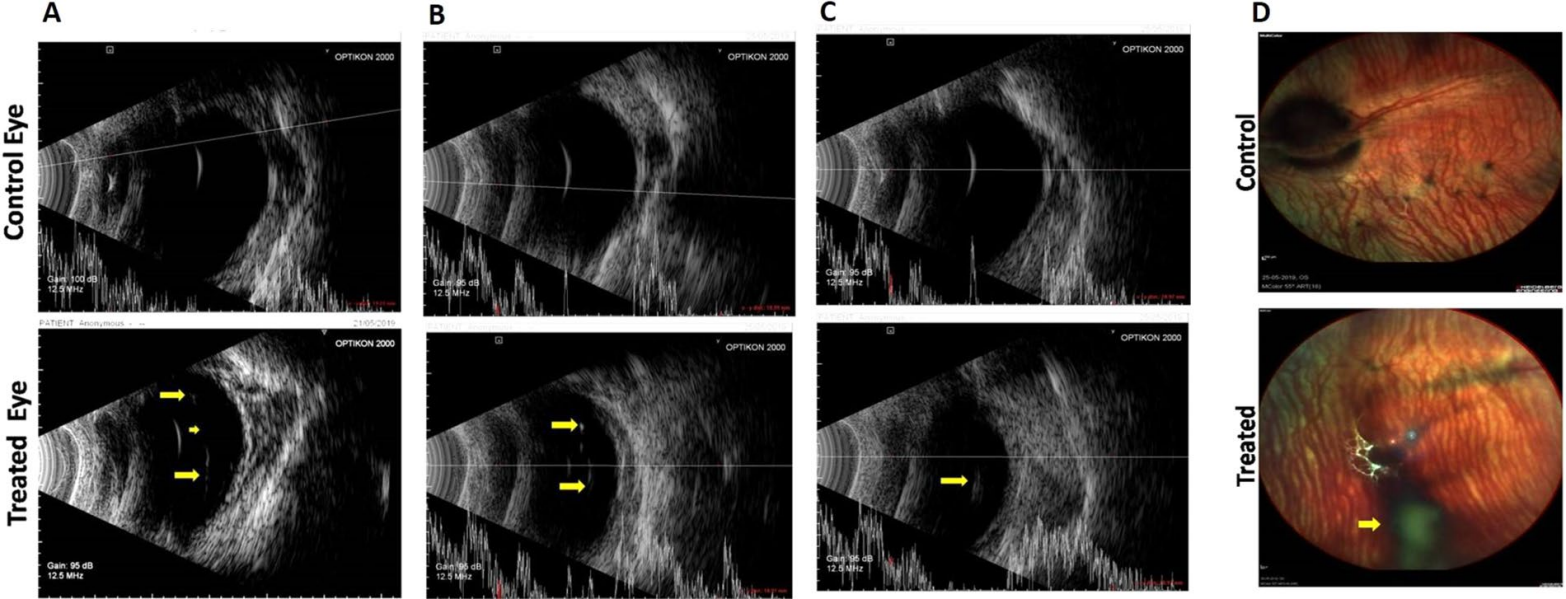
B-scan ultrasonography in three representative eyes following treatment with RCBD + CBD: Representative images of eyes treated with CBD and RCBD showing complete PVD (Panels A and B, marked by arrows), and partial PVD (Panel C), along with the corresponding contralateral eyes (controls). (Panel D) Fundus of representative rabbit eye with complete PVD, following the intravitreal injection of combination of CBD and RCBD; the posterior hyaloid membrane appears as a shadow in the fundus image which reflects PVD (marked with arrow).
